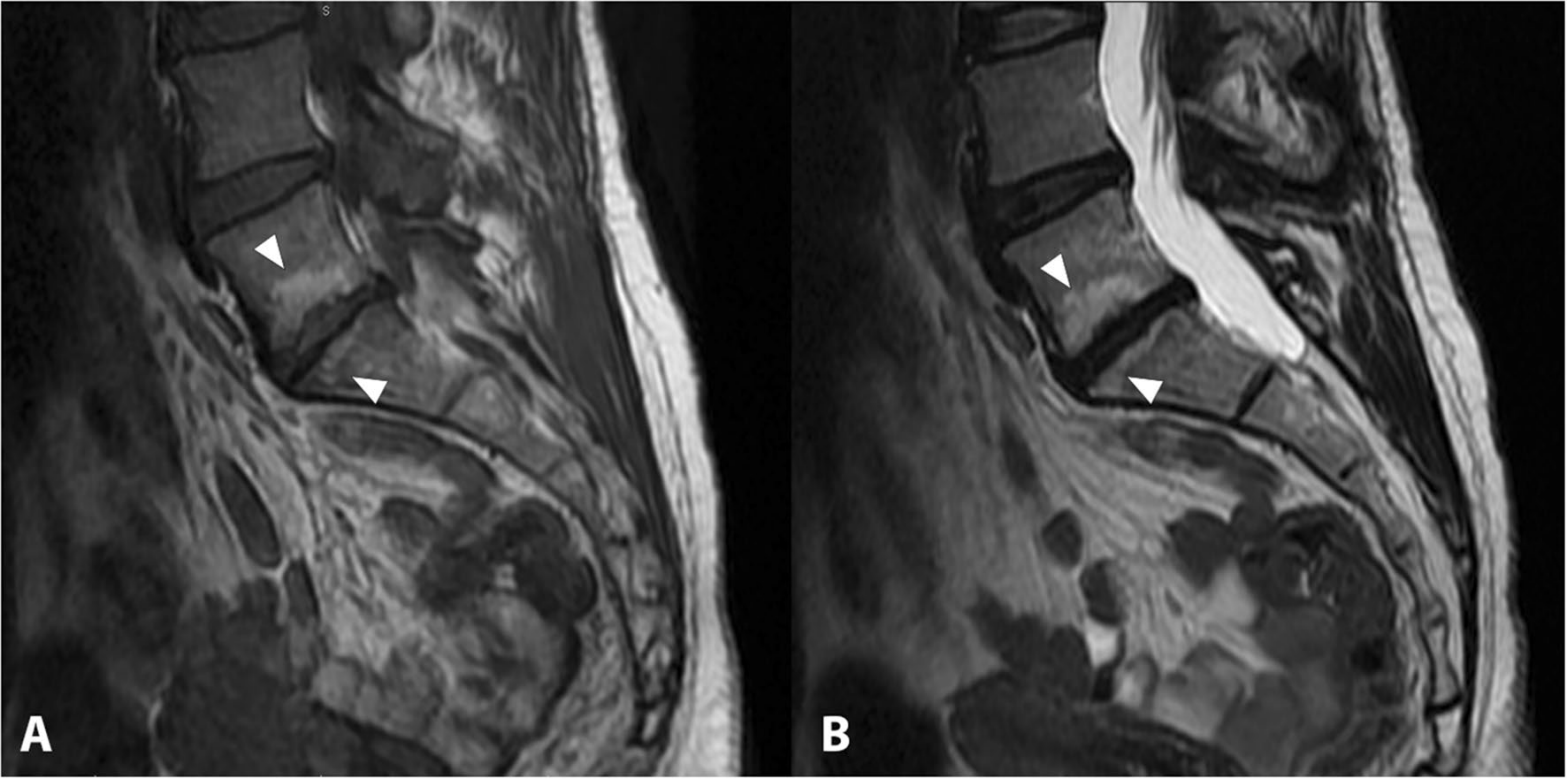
Sagittal T1-weighted image (a) and sagittal T2-weighted image (b) showing post-inflammatory area of fatty degeneration of the subchondral bone marrow of adjacent vertebral bodies L5/S1 (Modic type 2) (arrowheads)
